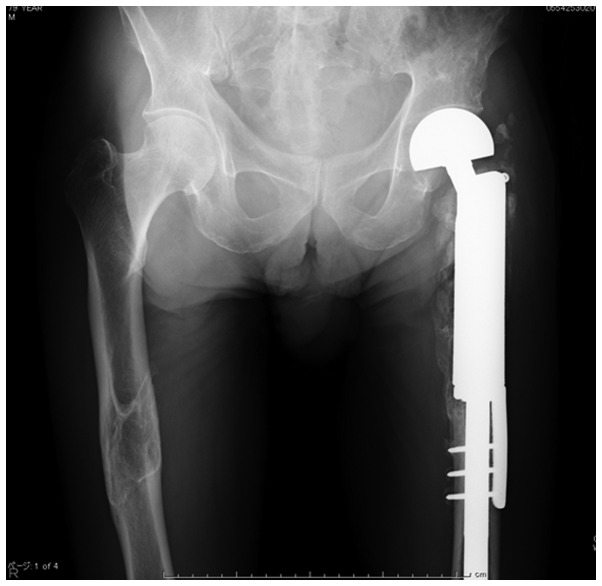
Case one: Radiographical observations following surgery. The metastatic bone lesion was resected and reconstructed using a tumor prosthesis.
